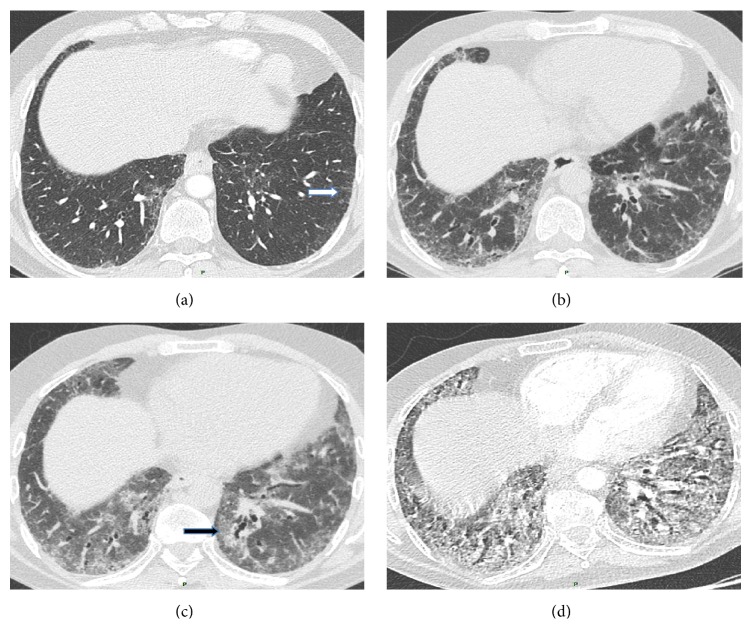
(a) HRCT in 2007 showed slight subpleural lines (arrow). (b) HRCT in March 2012 showed a predominance of subpleural reticular abnormalities with some ground glass features. (c) HRCT in July 2012 showed a worsening of reticular features with new ground glass opacities (arrow). (d) HRCT in August 2012 showed diffuse ground glass opacities with traction bronchiectasis.
